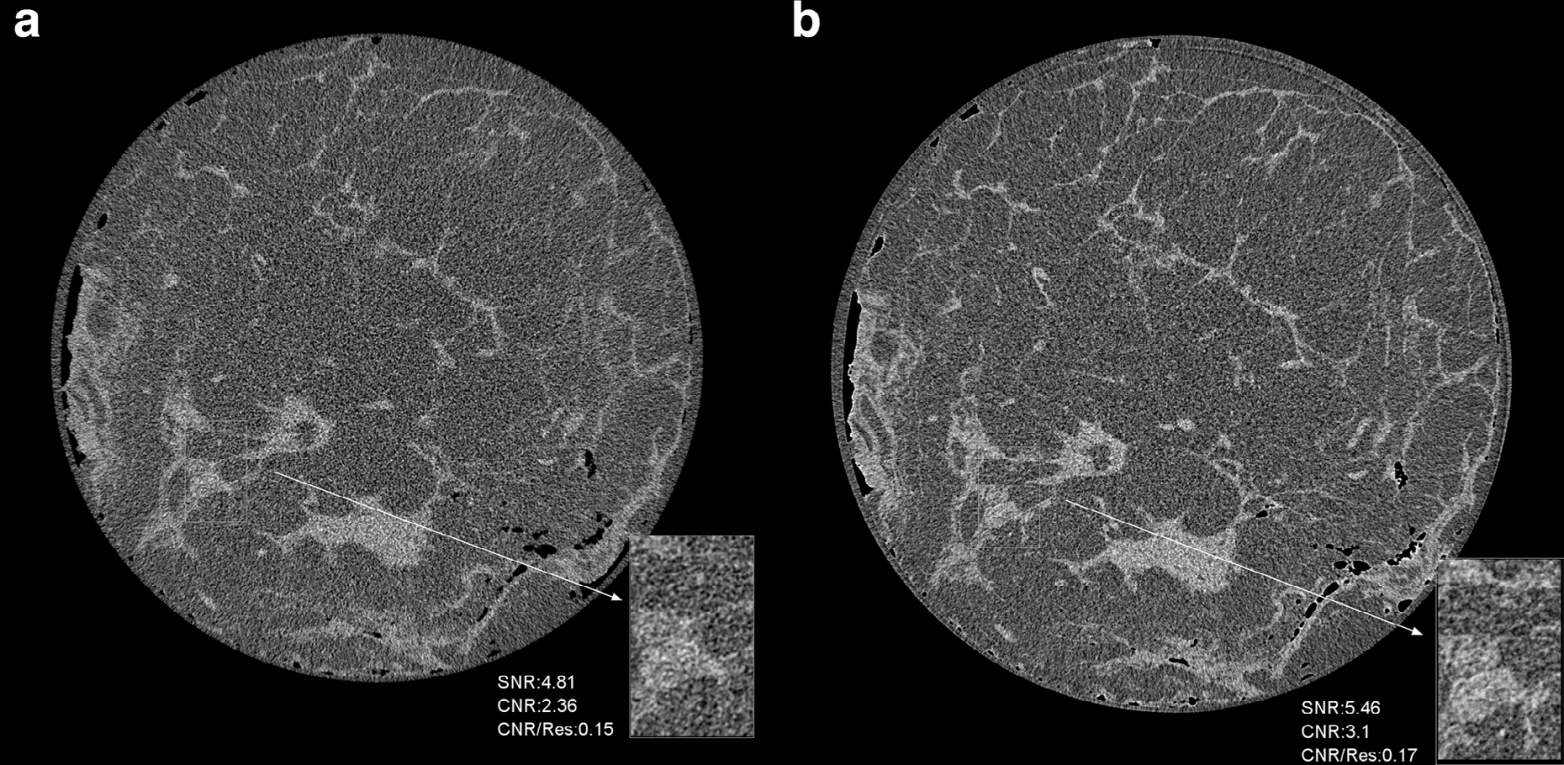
Comparison of Image Quality between PB-CT and AB-CT at the same radiation dose. (a) Image obtained with AB-CT at 32 keV and 4mGy. (b) Image obtained with PB-CT at 32 keV and 4 mGy, which demonstrates superior image quality. It should be noted that the PB-CT image exhibits a geometrical magnification factor of 1.04 due to the mastectomy sample being scanned at a 6 m object-to-detector distance, compared to the 0.19 m distance in the AB-CT image.
